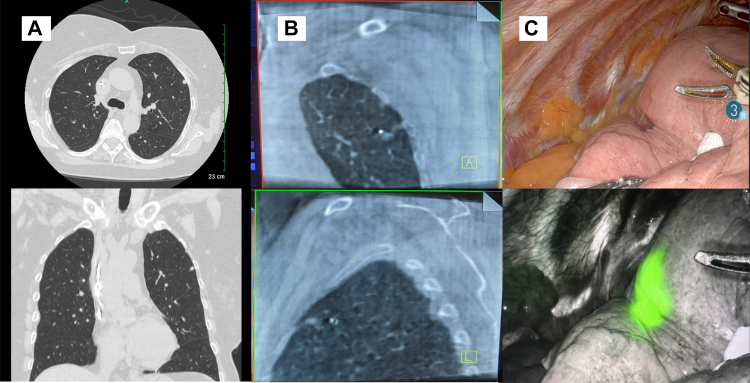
A-C, Representative imaging from indocyanine green-soaked fiducial marker (ICG-F) placement bronchoscopic procedure followed by robotic resection. A, Chest CT scan showing a 7-mm left upper lobe peripheral pulmonary lesion (PPL) in a patient with breast cancer and concern for metastatic disease. B, Cross-sectional imaging after ICG-F placement near the PPL via shape-sensing robotic-assisted bronchoscopy. C, Images from surgical resection showing absence of any visible indications of the PPL, lack of dye dispersion, and appropriate illumination of the target with the ICG-F marking when near-infrared imaging is used.
